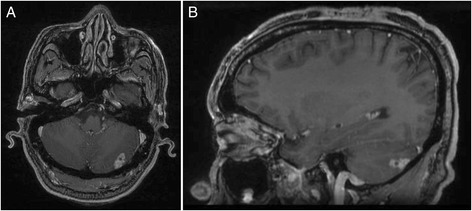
MRI of the central nervous system at diagnosis. Gadolinium enhanced T1 axial (a), and coronal (b) views. The images show an isolated left posterior cerebellar lesion consistent with an abscess
